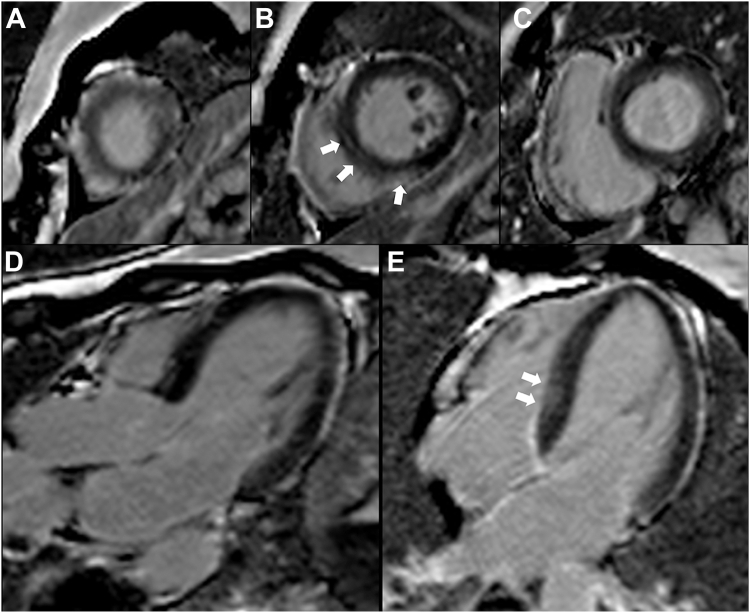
Late gadolinium enhancement sequence on cardiac magnetic resonance imaging showing possible focal mid wall (nonischemic) scar in the septum. (A-C) Short-axis view. (A) apical, (B) mid, and (C) basal segments. (D) Three-chamber view. (E) Four-chamber view.
